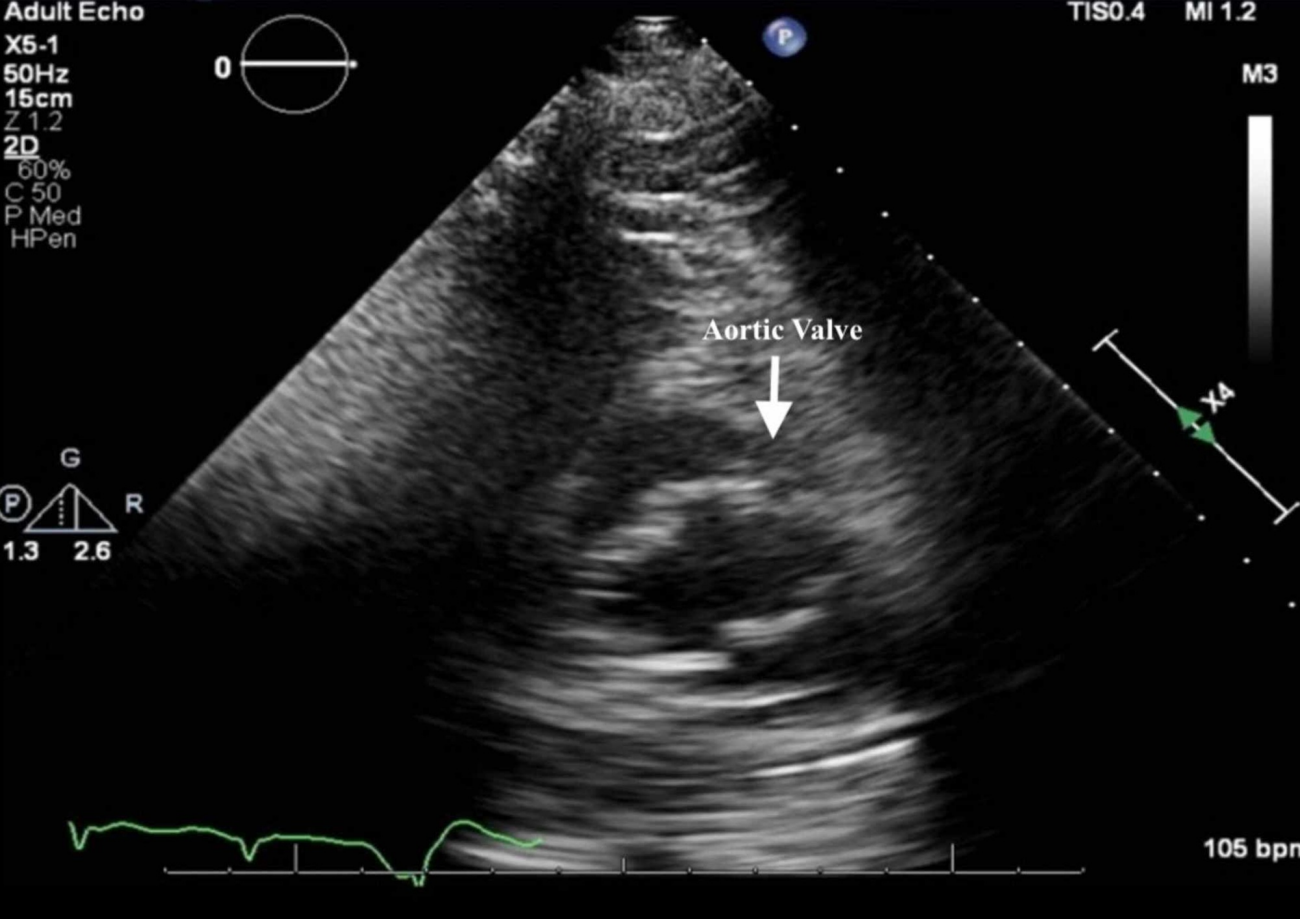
TTE short axis view demonstrating aortic stenosis. TTE, transthoracic echocardiogram
